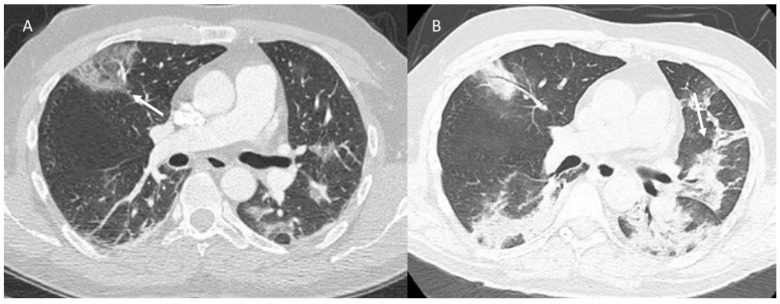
COVID-19 patient at peak stage. CT (axial plane: (A,B)) shows consolidation (arrow).
